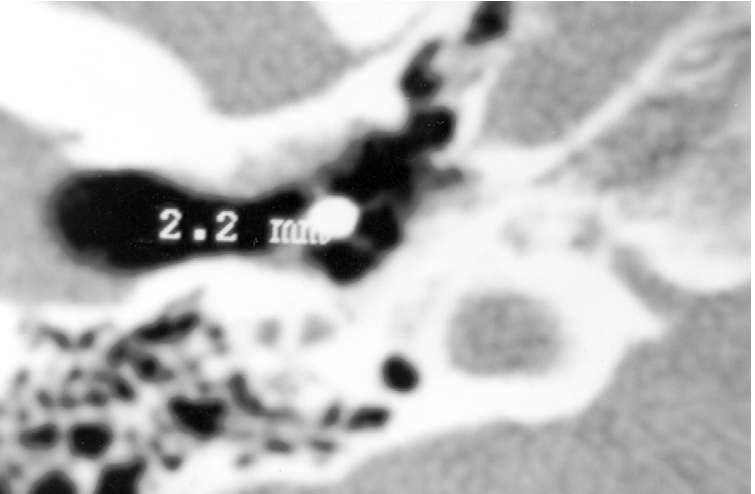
Coronal CT scan demonstrating a metallic foreign body in middle ear.
